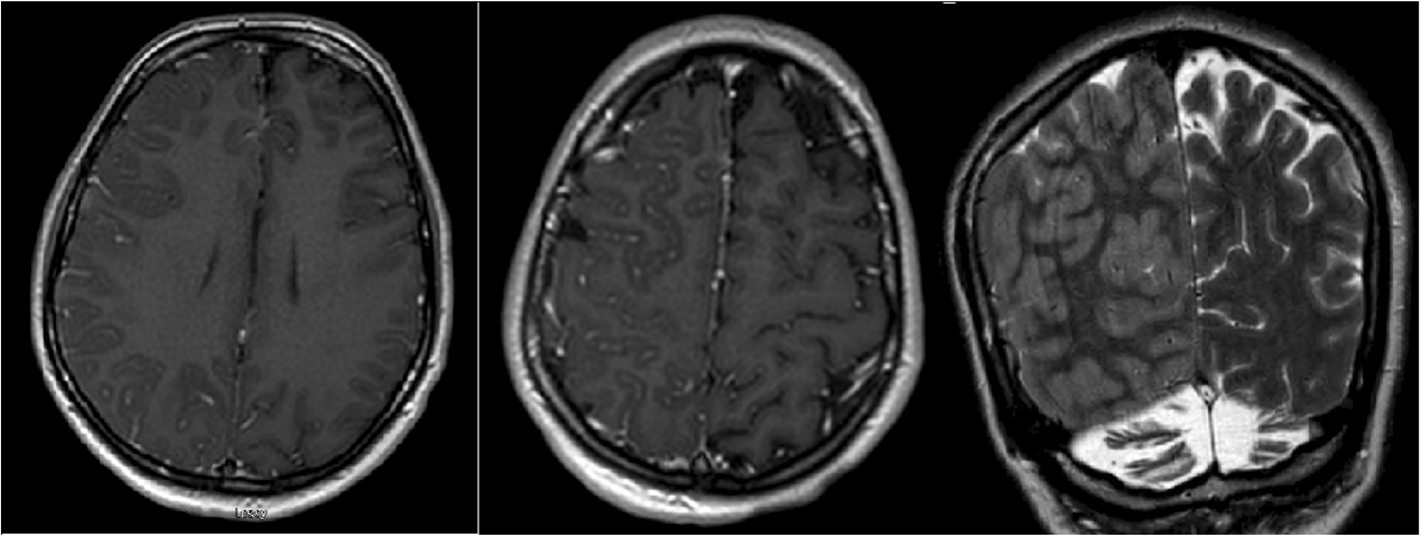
Magnetic resonance imaging scans showing right hemispheric leptomeningeal enhancement and edema in all three panels: T1-weighted sequence images with contrast in left and central panels and T2-weighted sequence image on right panel
